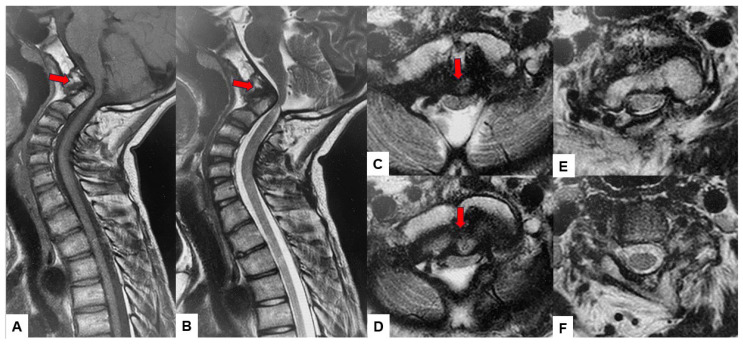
Preoperative MR imaging, (A) T1 weighted mid-sagittal MR imaging, (B) T2 weighted mid-sagittal MR imaging, (C) T2 weighted axial MR imaging at C1, (D) T2 weighted axial MR imaging at C1-2, (E) T2 weighted axial MR imaging at C2, (F) T2 weighted axial MR imaging at C3. The spinal cord was compressed severely due to basilar invagination. The red arrows show severe compression of the cervicomedullary cord by the dens.
